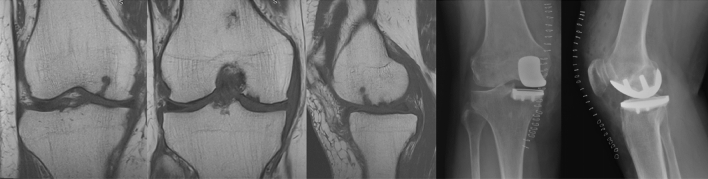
Magnetic resonance images, coronal and sagittal t1 scans show advanced medial compartment chondropathy. Postoperative radiographs after medial UKA, AP, and lateral view.
